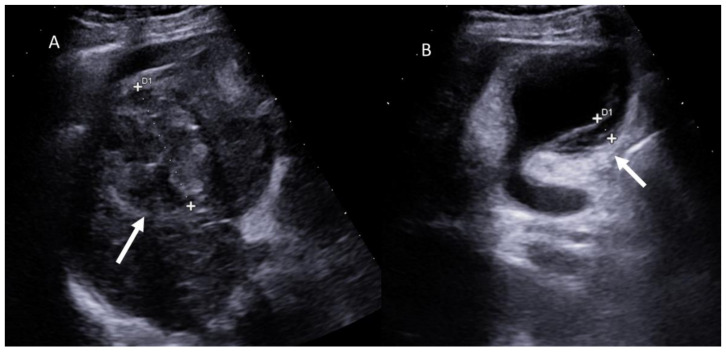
Ultrasound assessment of radio frequency treated HCC on V segment ((A) arrow). In (B) arrow shows cholecystitis.
